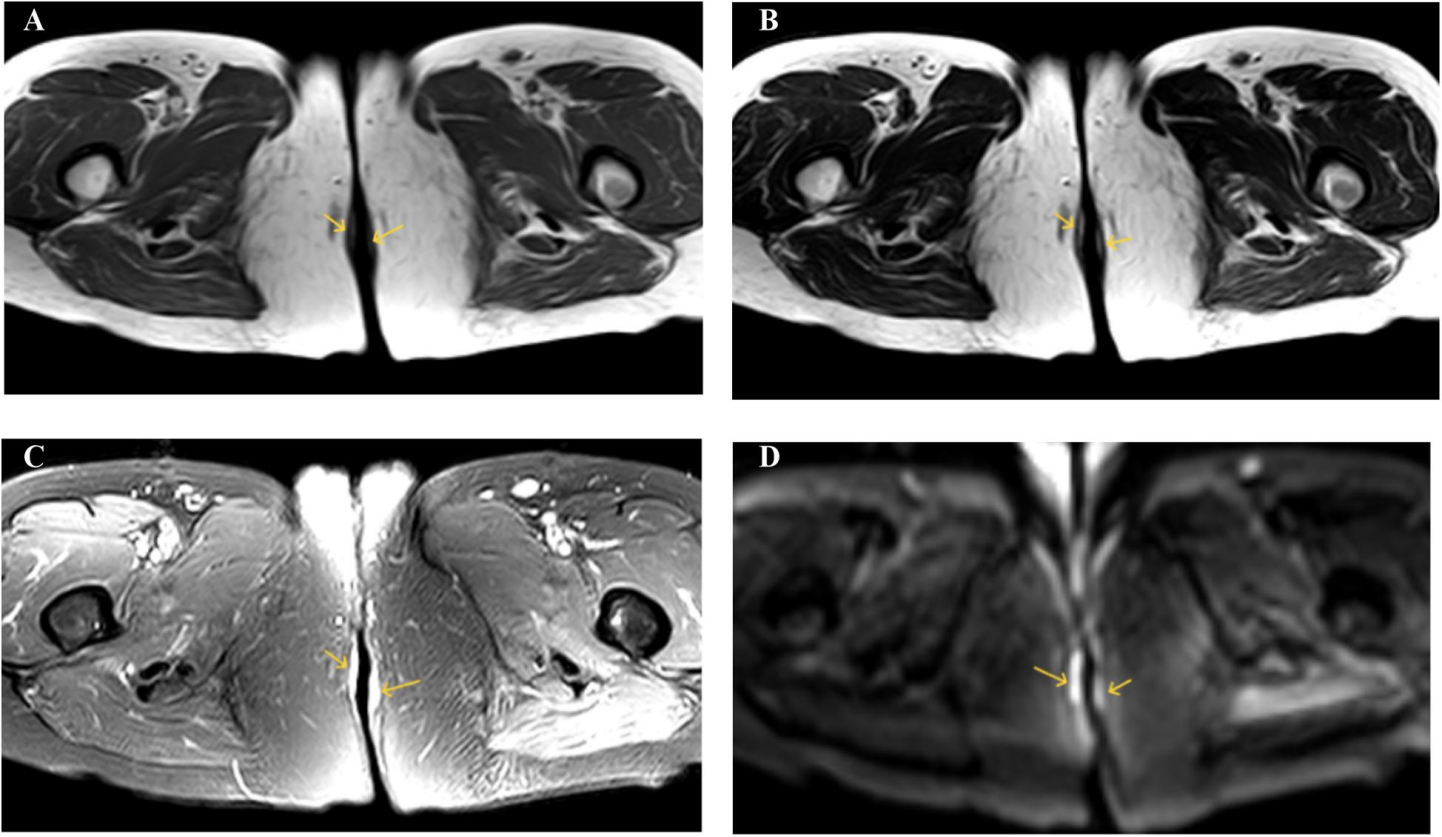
(A) T1WI shows low signal intensity. (B) T2WI shows high signal intensity. (C) SPAIR shows high signal intensity. (D) DWI shows high signal intensity.
